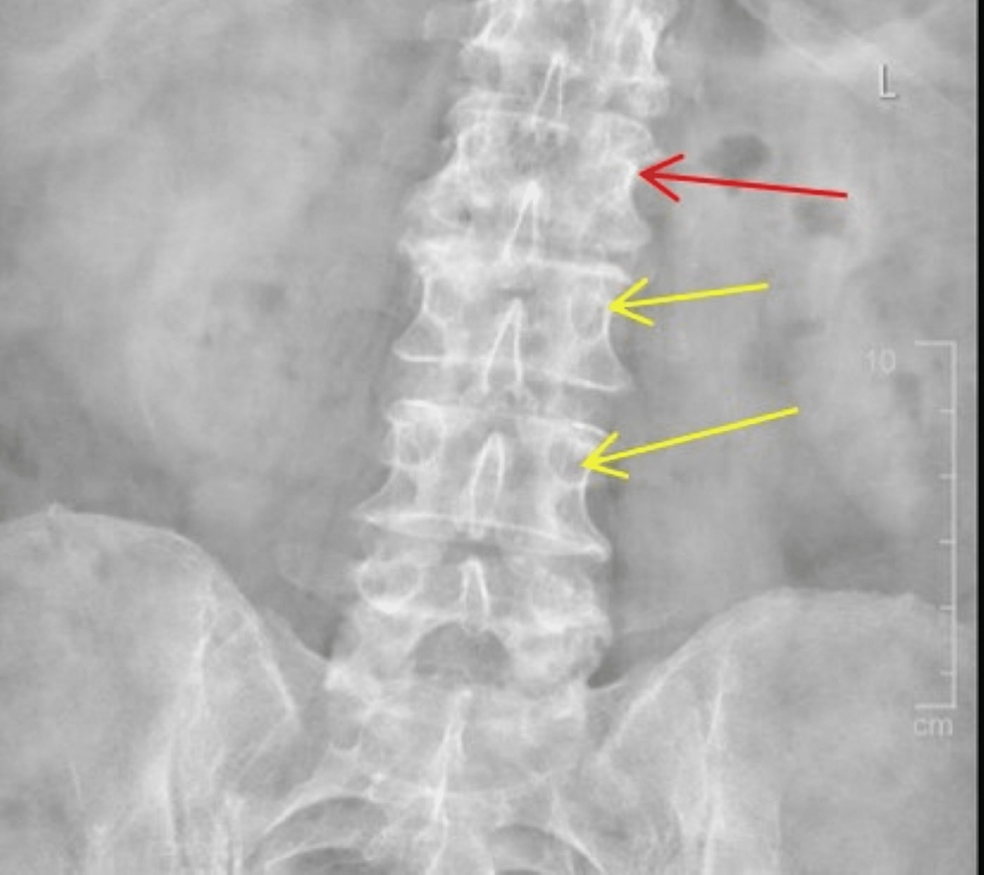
Frontal radiograph demonstrates an irregularity at the left L2 vertebral body pedicle with a wide zone of transition (red arrow) compared to the normal pedicle (yellow arrow).
